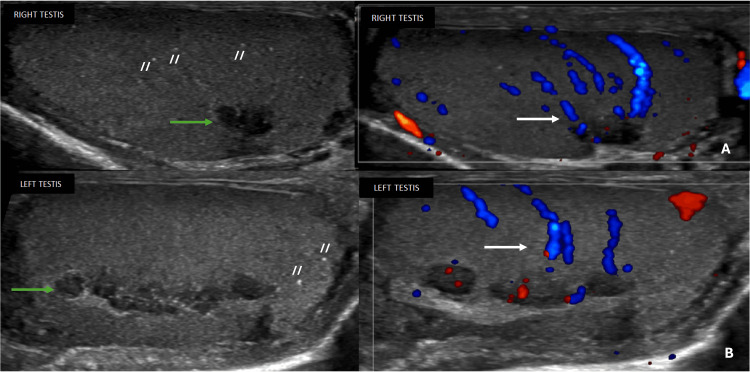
Scrotal ultrasound demonstrating early-stage (Stage II) TART with preserved testicular architecture. (A) Right testis showing preserved volume with multiple punctate intratesticular calcifications- microlithiasis (//) without diffuse parenchymal distortion. A small irregular hypoechoic lesion (green arrow) is noted adjacent to the mediastinum testis with minimal internal and peripheral vascularity on Doppler imaging (white arrow). (B) Left testis showing similar findings of preserved volume with intratesticular microlithiasis (//) and small hypoechoic mediastinal lesion (green arrow) with minimal vascularity (white arrow). No mass effect or vascular compression is seen. TART: testicular adrenal rest tumour
